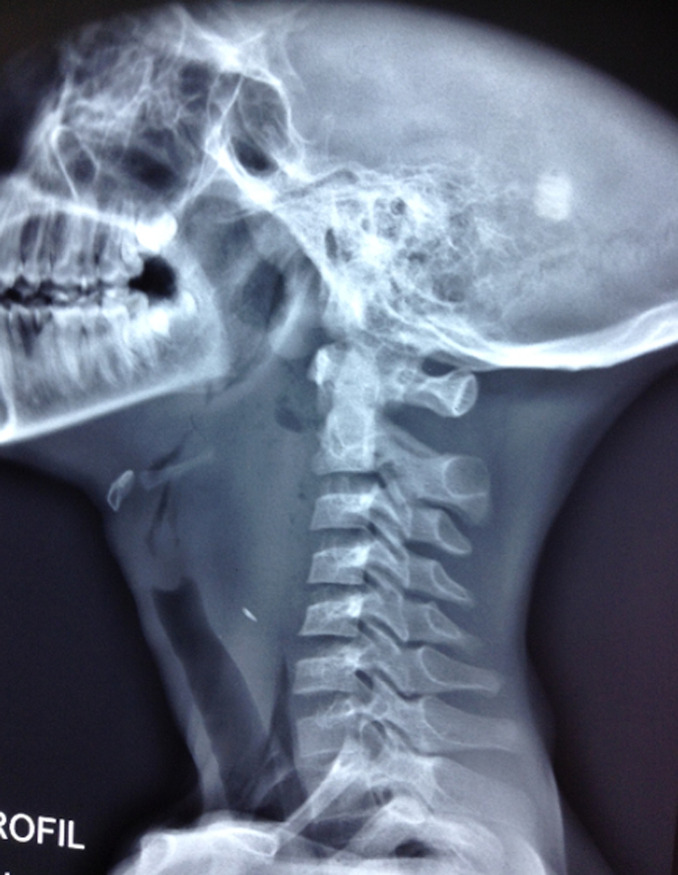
radiographie cervicale profil du 4^e^ patient, montrant une opacité surmontée d´un niveau hydroaérique avec le signe de Miningerode, sans corps étranger visible et obstruant considérablement les voies aériennes supérieures
